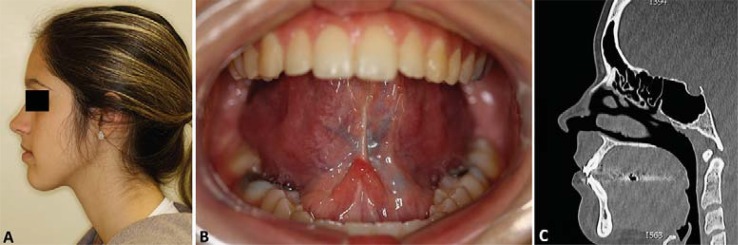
Clinical control during the five-year follow-up. A – Post-operative profile picture. B – Post-operative intra-oral clinical presentation. C – Sagittal computed tomographic (CT) image. After 5 years of follow-up, there was no recurrence of the lesion clinically, which was confirmed by CT scan
